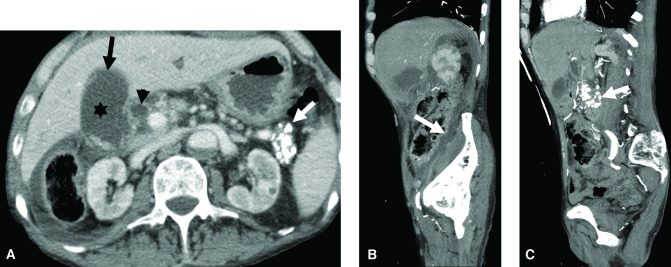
Abdominal CT pre cholecystectomy showing gangrenous cholecystitis. Axial (A) and sagittal (B, C) images demonstrating changes of acute gangrenous cholecystitis. There is a distended gallbladder with layering stones (asterisk, A), mural discontinuity in the fundal region (black arrow, A), and small pericholecystic fluid. There is a small amount of free fluid (arrow, B) extending from the right upper quadrant to the right lower quadrant without evidence of retroperitoneal abscess. Also note the intra- and extrahepatic (arrowhead, A) biliary dilation, and extensive pancreatic calcifications consistent with chronic pancreatitis (white arrow, A, C).
